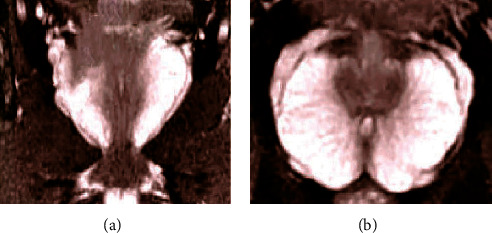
MRI images of normal prostate: (a) the coronal section and (b) the horizontal section.
